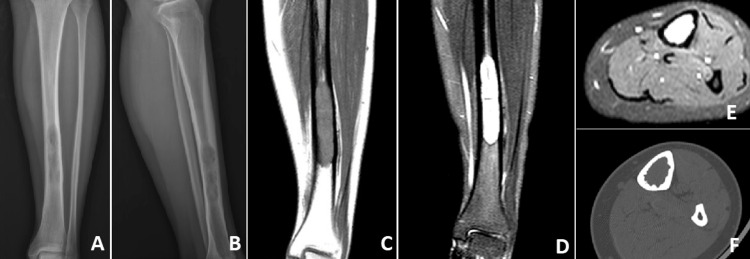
Preoperative images a) anterior-posterior radiograph, b) lateral direct radiograph, c) MRI T1 coronal section, d) MRI T2 coronal section, e) MRI T2 axial section, and f) axial tomography section MRI: Magnetic resonance imaging
